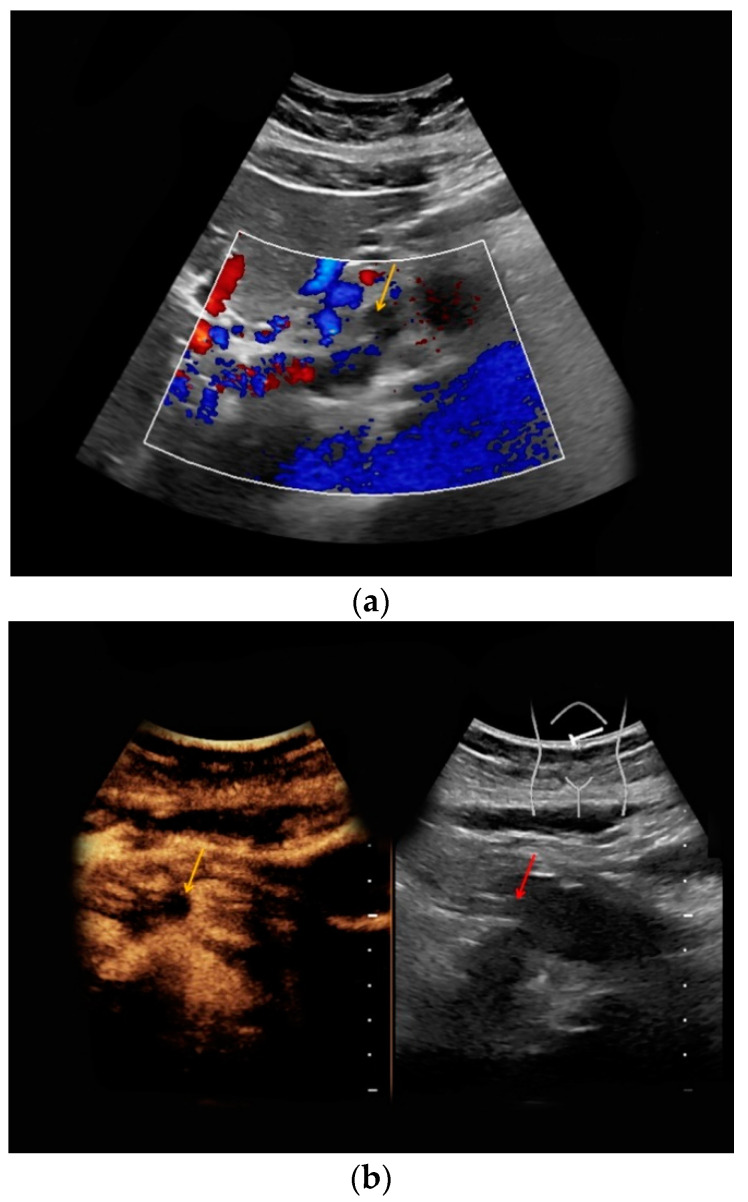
Superior mesenteric vein thrombosis, 14 weeks of pregnancy. (a) No internal Doppler signal in the superior mesenteric vein in Doppler mode (yellow arrow). (b) Upon intravenous contrast application, only very few microbubbles were marginally detected in the superior mesenteric vein due to the thrombosis (yellow arrow).
